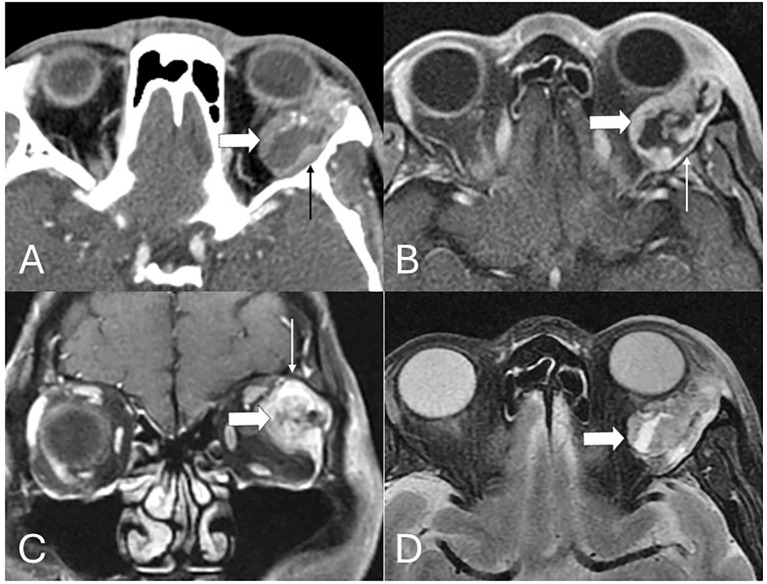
A 57-year-old man with a left lacrimal pleomorphic adenoma. (A) Axial CT with contrast shows a cystic and solid lacrimal mass with calcification (white arrow). Note the remodeling of the lateral orbital wall (small black arrow). (B, C) Axial and coronal T1 post-contrast MRI shows an oval-shaped mass with heterogeneous enhancement (large white arrows) and remodeling of the orbital wall and roof, respectively (small white arrows). (D) Axial T2 MRI shows a cystic and solid appearance of the lacrimal mass (arrow).
